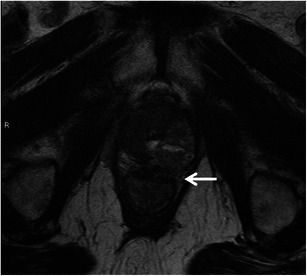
Axial T2-weighted MRI reveals extracapsular extension of prostate cancer with obliteration of the left rectoprostatic angle (*arrow*) and soft tissue mass extending to the anterior serosal surface of the rectum
